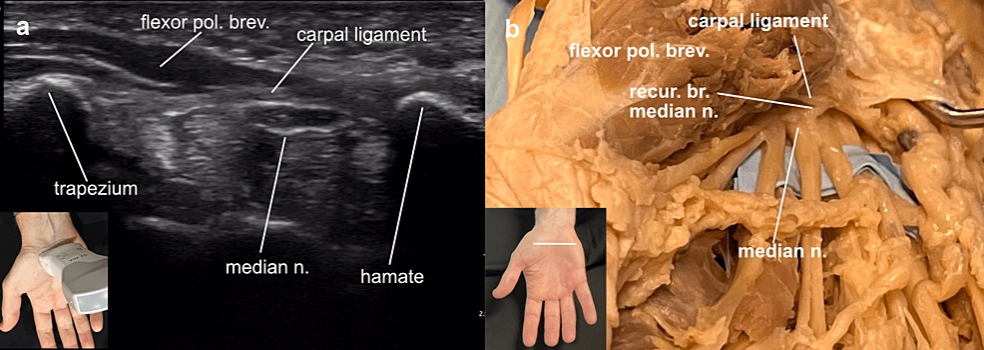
Transverse carpal ligament Transverse carpal ligament: (a) ultrasound view of the right hand with the probe marker directed toward the patient’s right (the thumb) revealed the transverse carpal ligament between the trapezium and hamate bones, (b) cadaver view looking into the carpal tunnel from the palm, demonstrating the transverse carpal ligament, the median nerve with its recurrent motor branch, and the flexor pollicis brevis muscle overlying the ligament.
